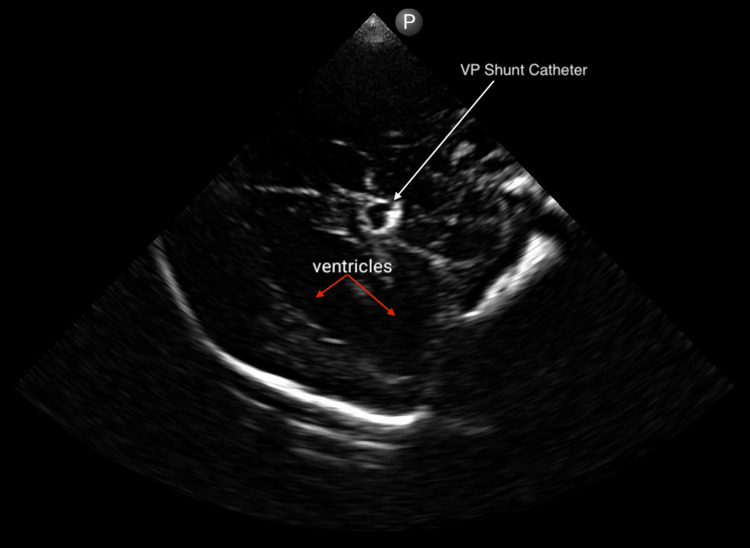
Point-of-care transcranial ultrasound obtained through a previous hemicraniectomy window demonstrating the ventricular system. The ventricles are visualized as anechoic structures (red arrows), allowing direct intracranial assessment. The ventriculoperitoneal shunt catheter is visualized as a hyperechoic linear structure (white arrow) within the ventricular system.
